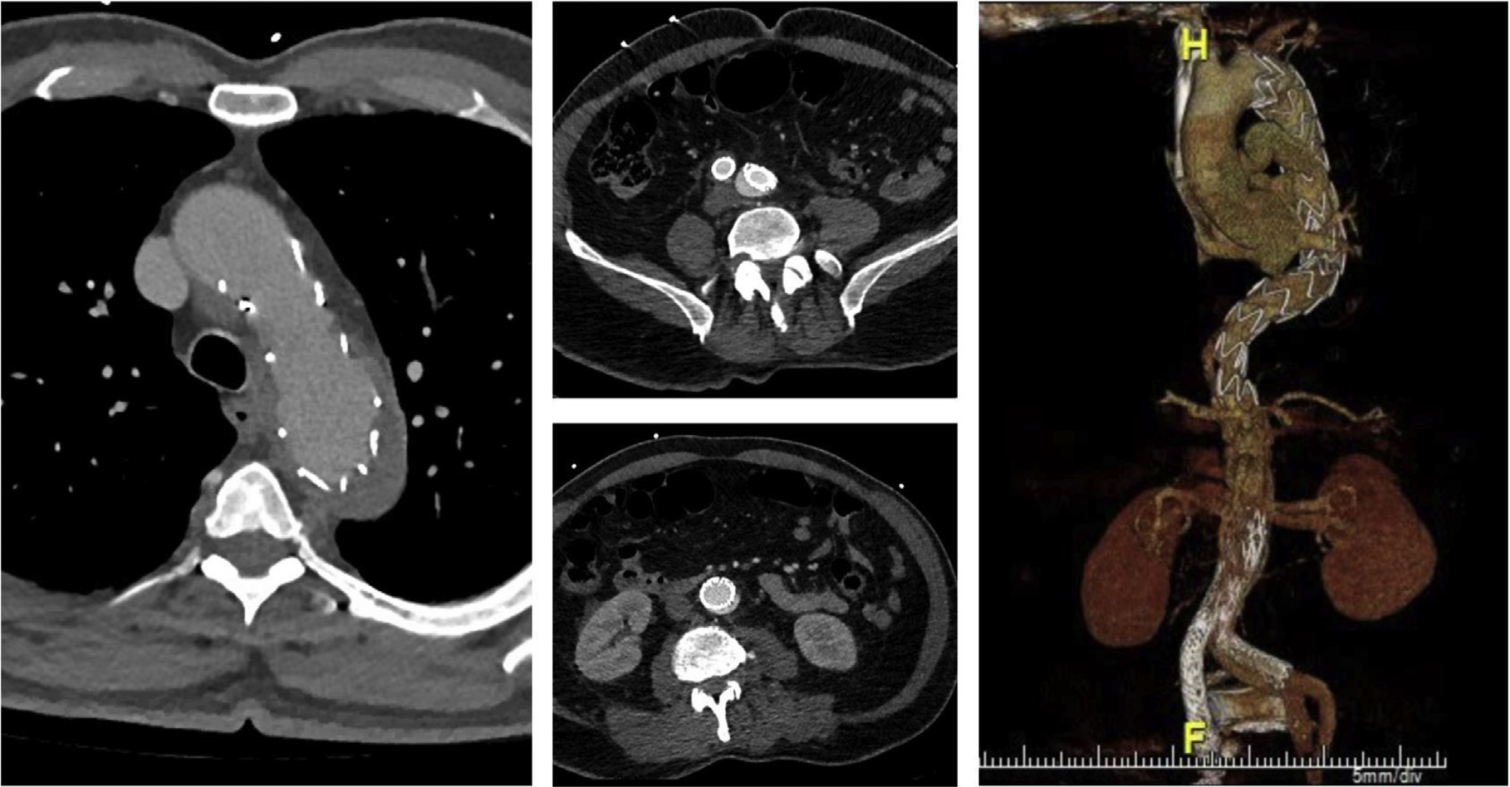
Postoperative computed tomography angiography shows remodeling of the thoracic aorta and reexpansion of the infrarenal endograft with reinforcement from an aortic cuff. Sufficient perfusion to the visceral and iliac arteries is visualized.
